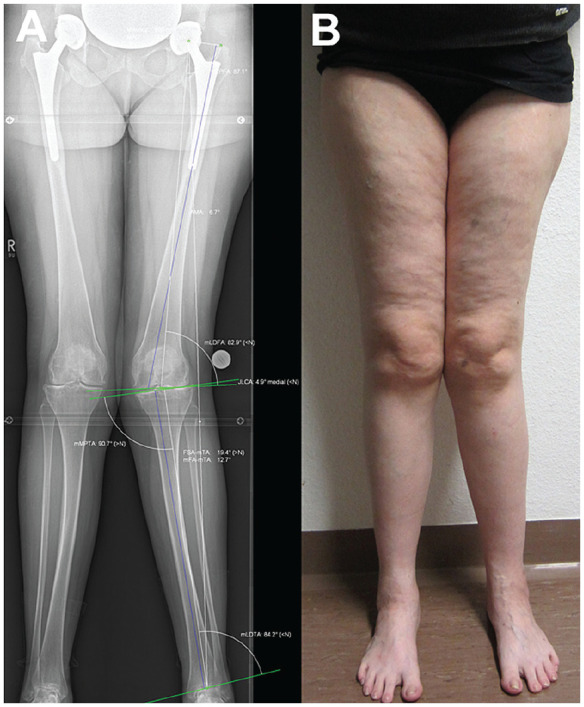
Case example: 55-year-old female patient with lateral knee pain. (A) Preoperative radiograph (deformity analysis: mechanical tibiofemoral angle [mTFA], 12.7°; mechanical lateral distal femoral angle [mLDFA], 82.9°; mechanical medial proximal tibial angle [mMPTA], 90.7°). (B) Clinical photograph. JLCA: joint line convergence angle.
